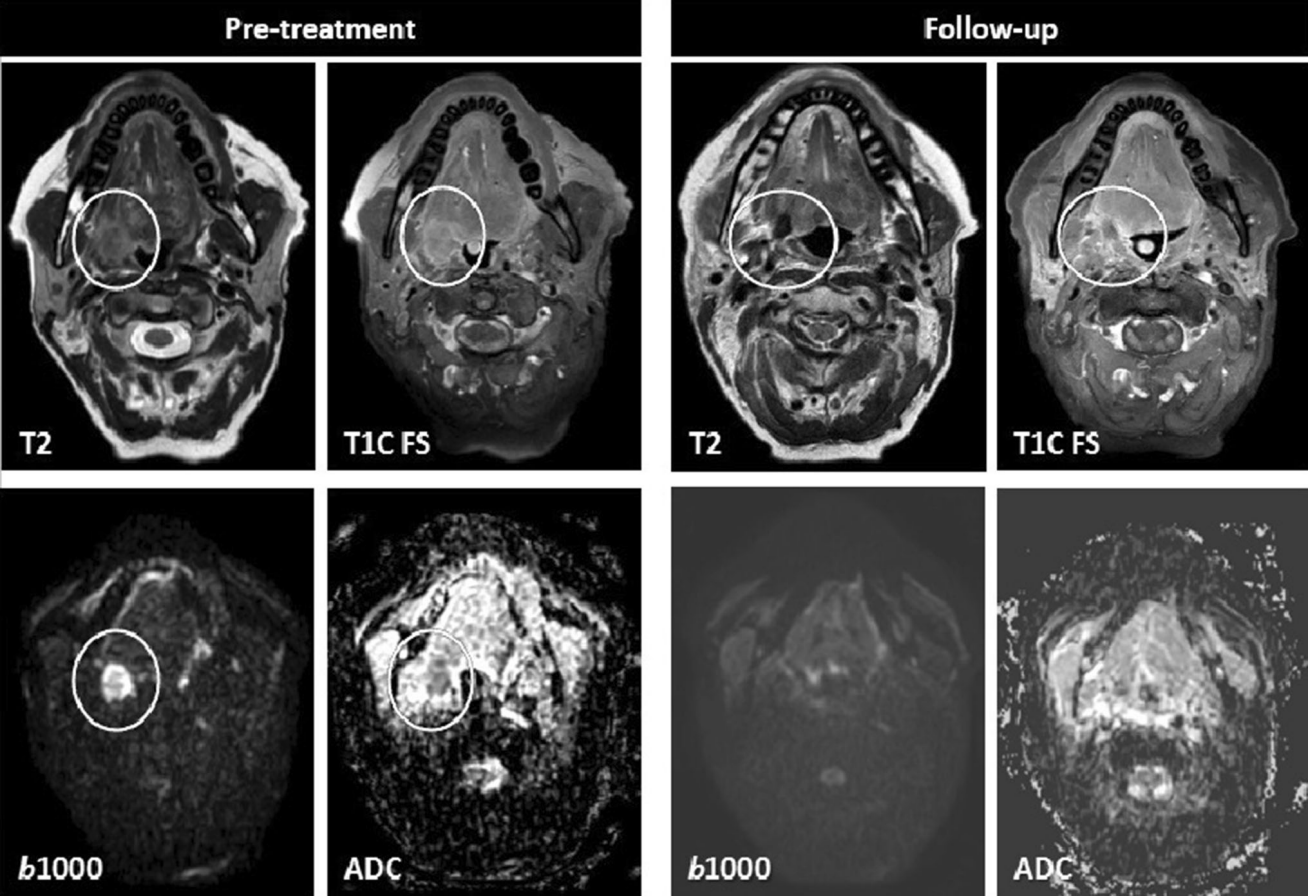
Tumor response confirmed on diffusion. A 54-year-old patient with a tumor at the retromolar trigonum showing high T2 signal, enhancement and diffusion restriction before treatment. Follow-up 6 months after radiation therapy showed at least partial response on anatomical MRI with some residual high T2 signal and enhancement. Diffusion restriction aided in the differentiation between residual tumor and post-therapy inflammation. Lack of diffusion restriction in this patient was in keeping with post-therapy changes
